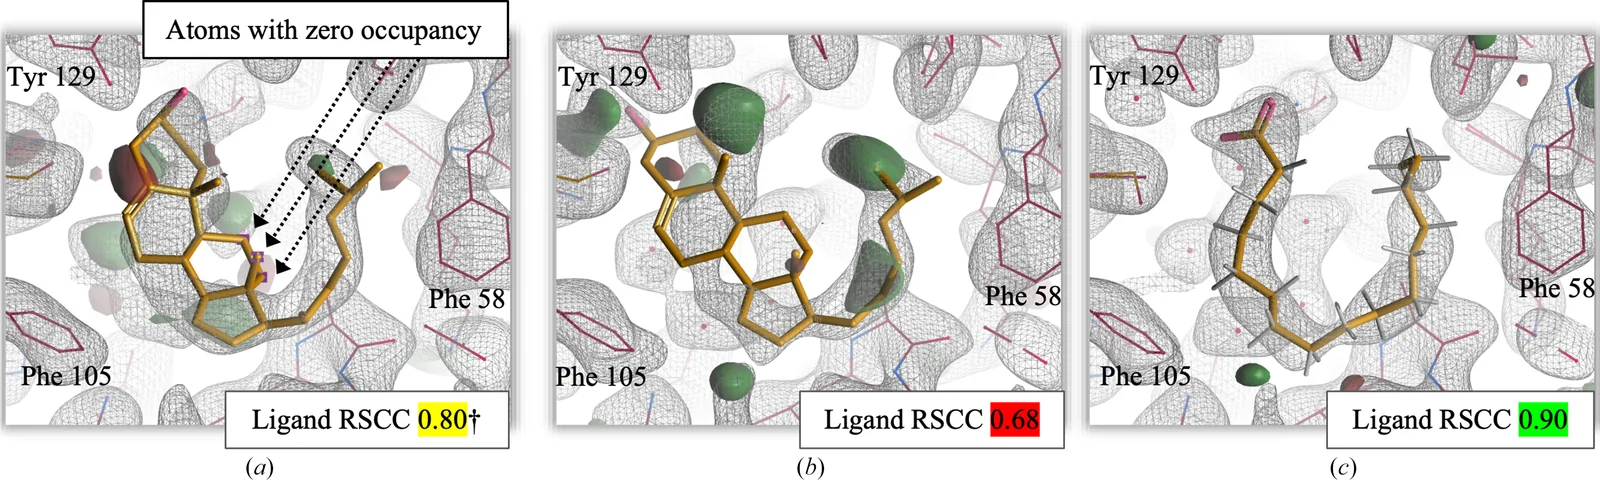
The cholesterol ligand of interest in PDB entry 8ivl has been fitted into electron density that is better explained by an endogenous fatty acid. (*a*) The cholesterol ligand in the PDB deposition is a poor fit to the electron density and has a strained conformation. † Note that the three atoms with zero occupancy do not contribute to the X-ray map calculation and consequently the RSCC is improved. (*b*) Refinement after *Coot* was used to interactively refit the cholesterol molecule to improve its geometry; this resulted in a worse fit to the electron density. (*c*) Modelling the ligand as the anion of a stearate fatty acid results in a much better density fit and in improved ligand-validation metrics. Figure produced using *Coot* with the *BUSTER* 2*mF*_o_ − *DF*_c_ map contoured at 0.8 r.m.s.d. shown as a grey mesh and the *mF*_o_ − *DF*_c_ difference maps contoured at 3.0 r.m.s.d. shown as red (negative) and green (positive) solid surfaces.
